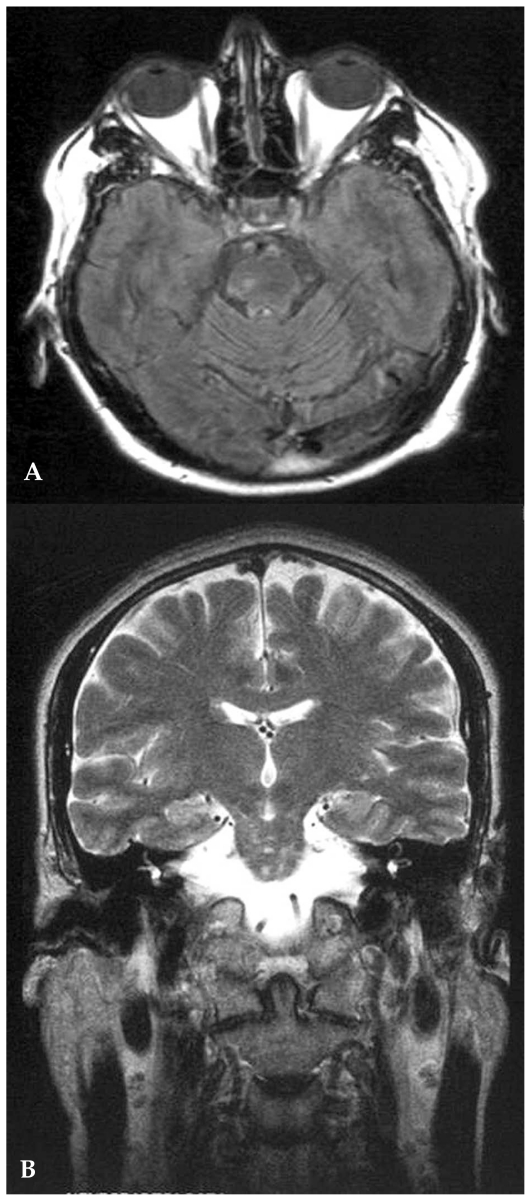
Behçet‘s disease, meningo-parenchymatous form: pontine lesions on axial (A) and coronal (B) T2-weighted MRI images. ultrasonography shows orbital and ocular hemodynamic alterations.
